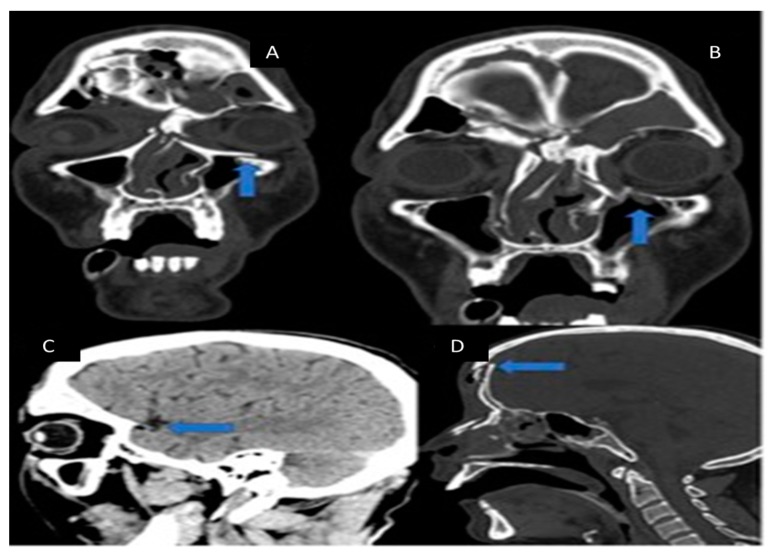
(A) CT image, coronal section—a telescoping fracture of the left inferior margin can be observed; (B) right inferior muscle incarceration in the fracture focus; (C) CT image, sagittal section—minimal pneumocephalus; (D) displaced posterior frontal sinus wall fracture.
